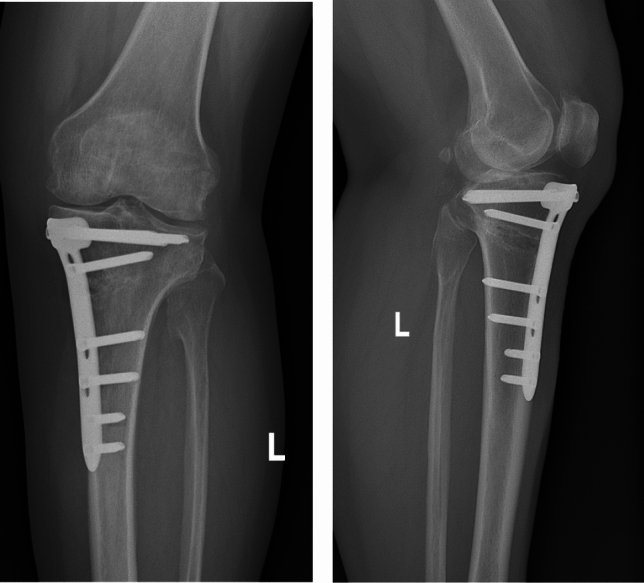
Positive and lateral X-ray films of the knee joint showing changes after high osteotomy and internal fixation of the left tibia, and the osteotomy surface and internal fixation position were satisfactory
